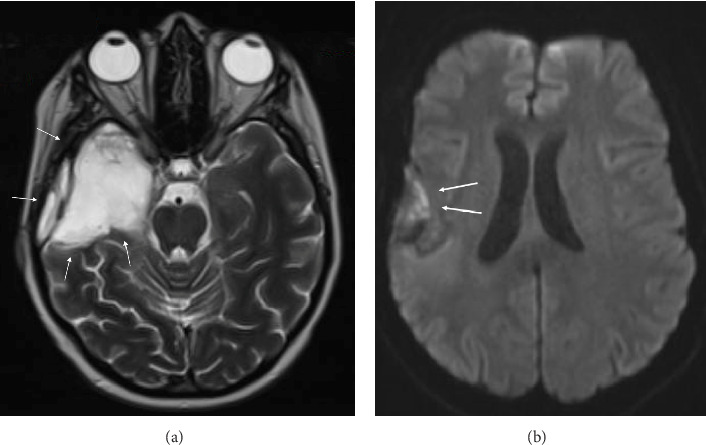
Six-month follow-up MRI head, demonstrating (a) no evidence of recurrence of the lesion on T2 sequence (denoted by arrows) and (b) improvement of diffusion restriction along the right MCA territory with minimal remaining signal (again, denoted by arrows).
